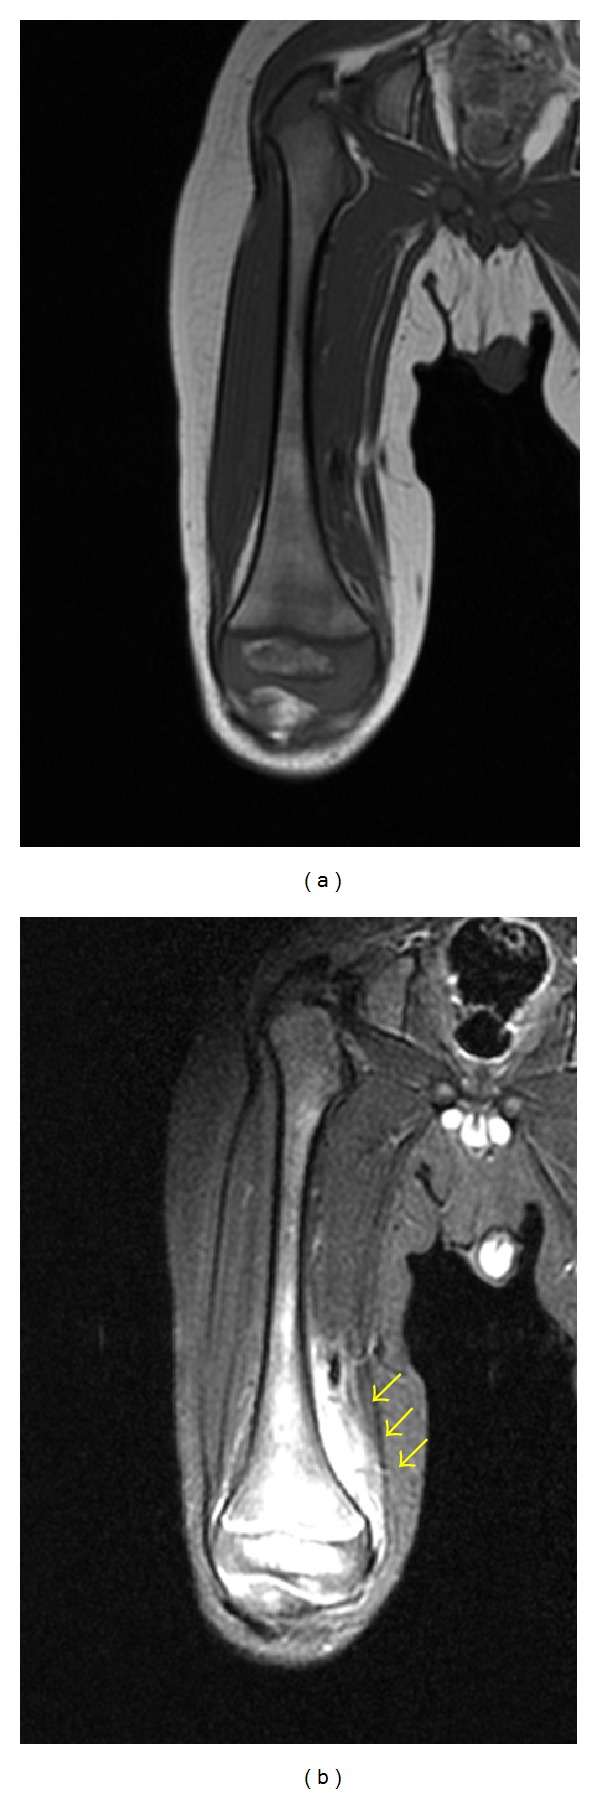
(a) Coronal T1-weighted image of the right femur shows hypointensity in the bone marrow of femoral diaphysis and epiphysis. (b) Coronal STIR sequence shows hyperintensity in the bone marrow of the femoral diaphysis and epiphysis, including the area around the soft tissue.
